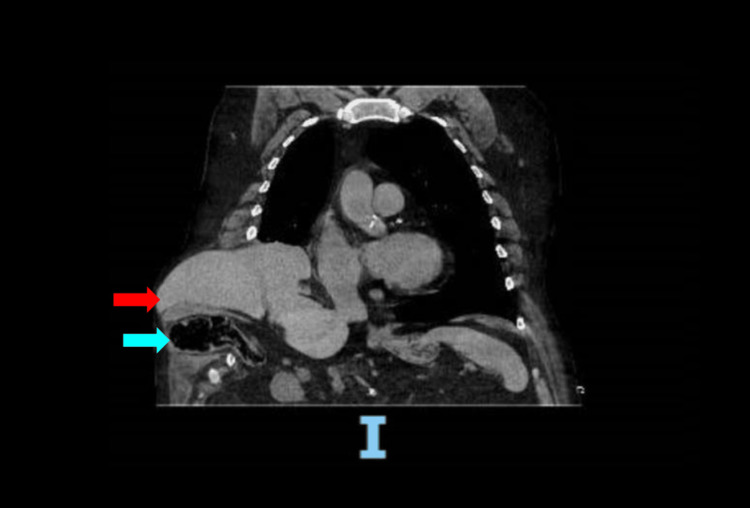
Coronal computed tomography image showing increased herniation of the right hepatic lobe and new herniation of nonobstructed right colon through the right seventh intercostal space. Red arrow: Right lobe of the liver; Blue arrow: Large bowel segment.
